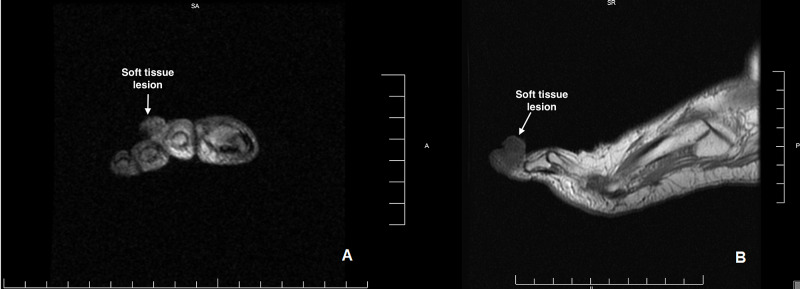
MRI of the soft tissue mass (arrow)
